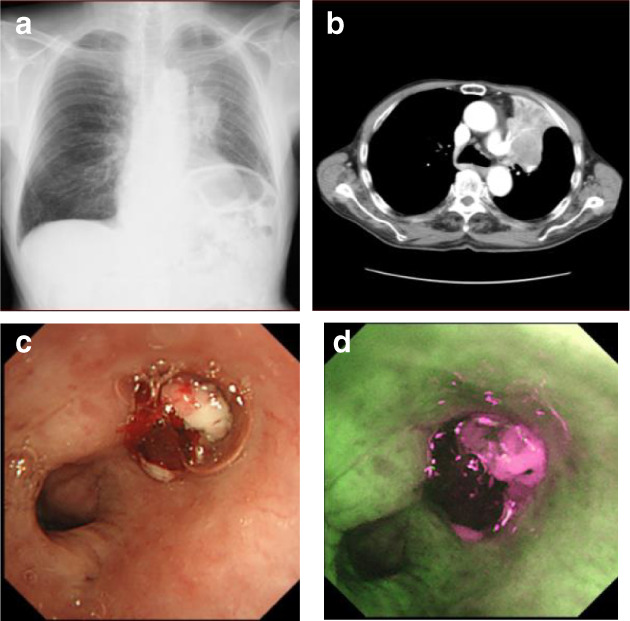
(a) Chest X‐ray and (b) contrast‐enhanced computed tomography (CT) at first admission. Bronchoscopy with (c) standard and (d) fluorescent observations revealed a tumor obstructing the left upper lobe.
